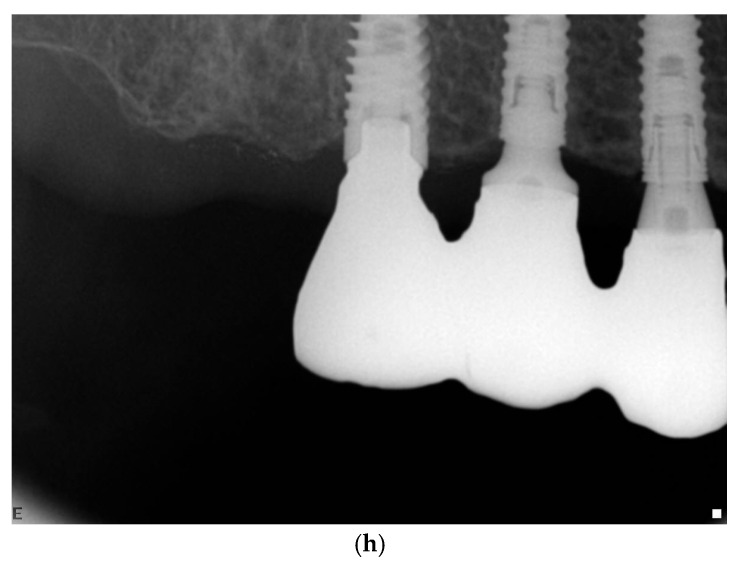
(a–h) Maxilla: (a) Two implants replacing missing right first and second premolars in an 83-year female. The implants shoulder was flushed with the bone. (b) Multiunit (test) and healing (control) abutments were connected after final seating of the implants. (c) Twenty-four weeks after placement, healthy peri-implant keratinized gingiva. (d) Healthy sulcular epithelium at both test (second premolar) and control (first premolar). (e) Transfer adaptation for “open tray “impression technique. (f) Radiographic transfer adaptation. (g) Final crowns at the 3-year follow-up. Opened embrasure spaces for enhanced interproximal plaque control. (h) Radiographic evaluation at the last follow-up (3-year) presents stable crestal bone level in both test and control sites.
